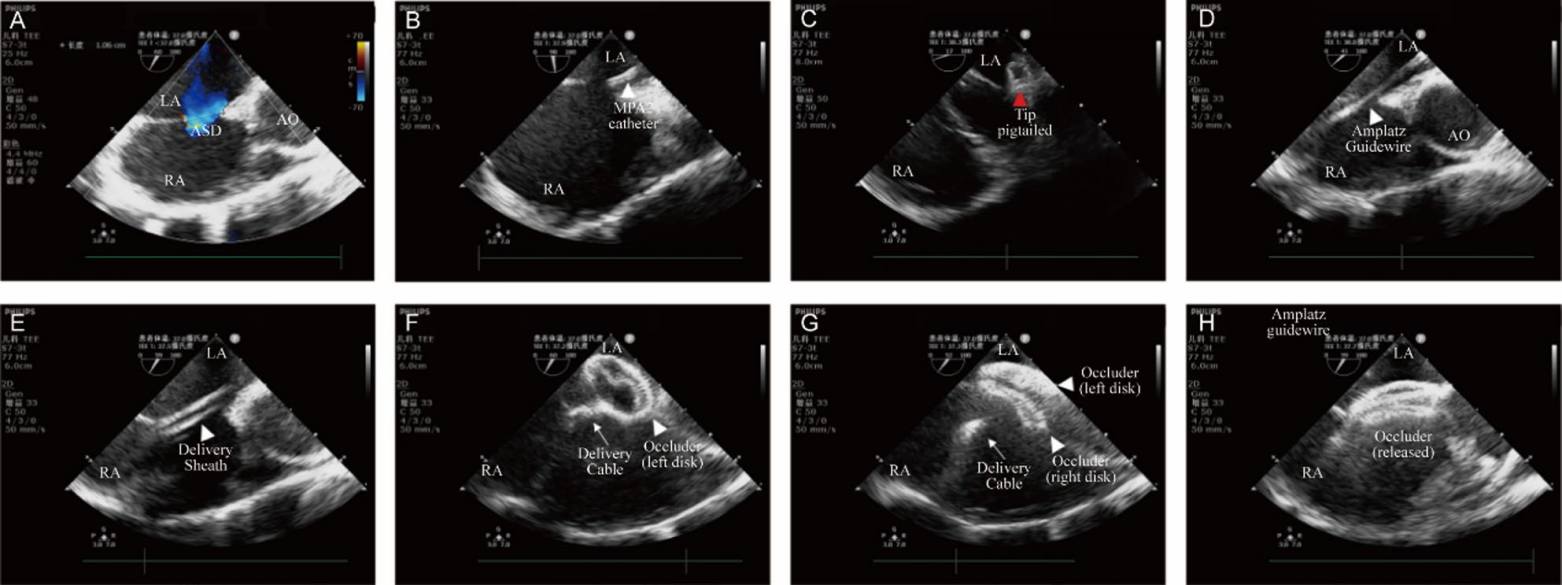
Transesophageal Echocardiography (TEE)-Guided Percutaneous ASD Closure Procedure. A Pre-procedural TEE assessment of the ASD diameter and rims. B The MPA2 catheter is advanced through the defect into the left atrium (LA). C The Amplatz super-stiff guidewire is advanced, with its tip curling into a “pigtail” shape (red arrowhead) within the LA to prevent wall injury. D The stiffened guidewire traverses the ASD, establishing a stable rail. E The delivery sheath is advanced over the wire; the parallel hyperechoic walls of the sheath and the inner wire create the characteristic “double-track sign” (white arrowhead), confirming correct trans-septal positioning. F Deployment of the left atrial disc. G Deployment of the right atrial disc. H Final release of the occluder after confirming stability. LA: Left Atrium; RA: Right Atrium; Ao: Aorta
